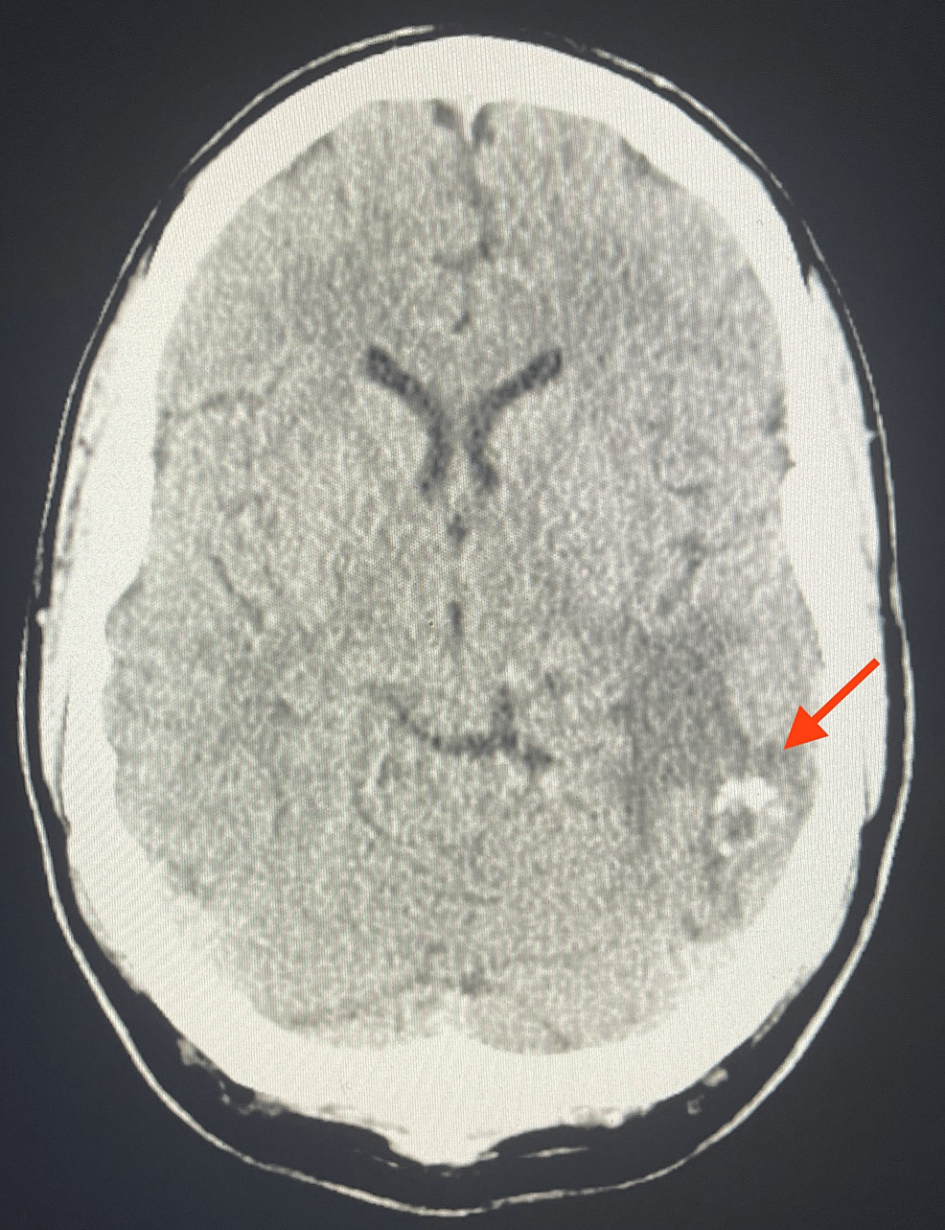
Non-contrast CT performed during admission The non-contrast CT shows a left temporal lobe AVM (red arrow) with no signs of acute infarcts, acute intracranial hemorrhage, and mass.
